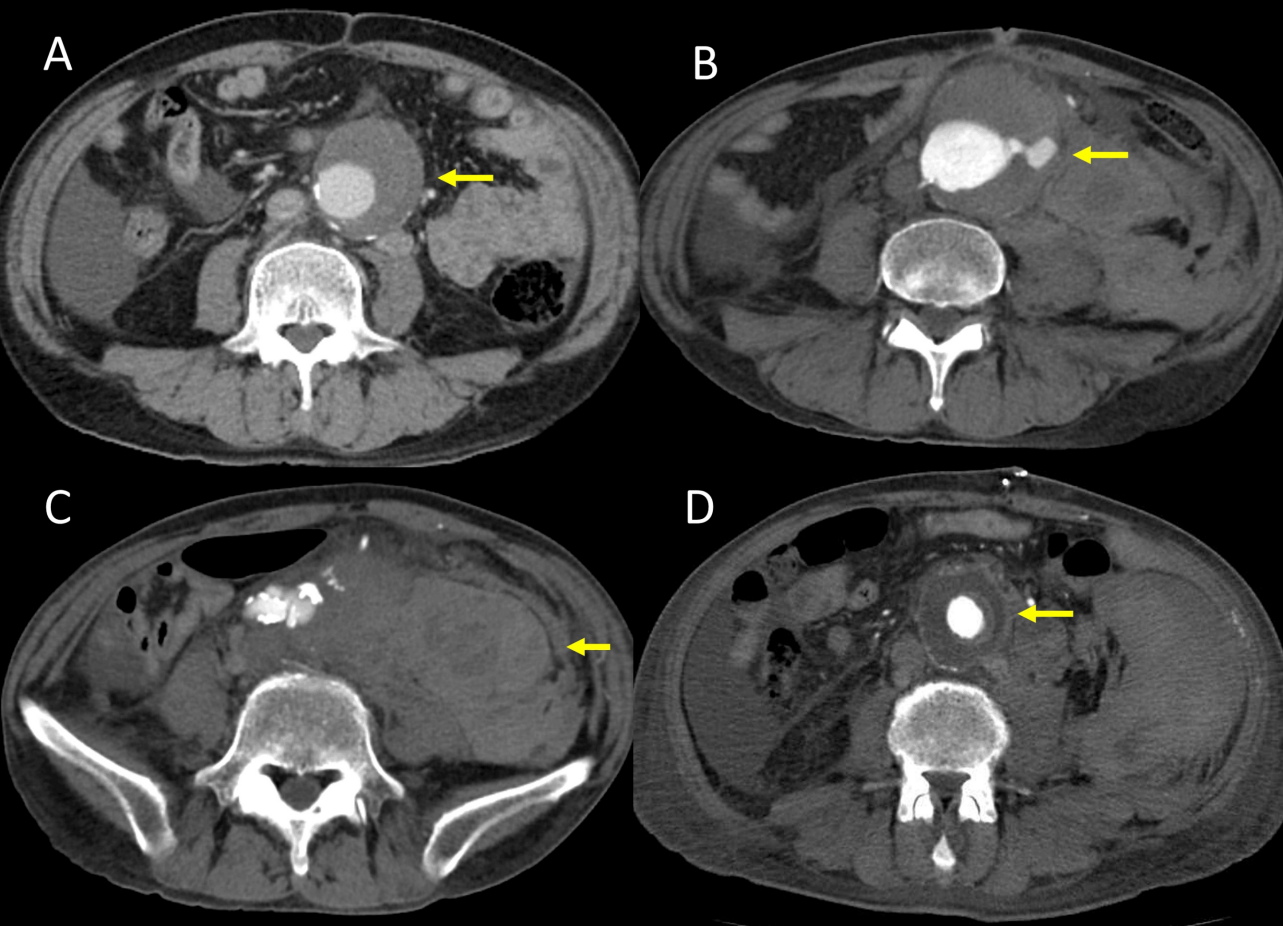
Comparison of pre-treatment and post-rupture axial CT findings. (A) Pre-treatment axial CT image showing an infrarenal abdominal aortic aneurysm measuring 52 mm. (B) Axial CT image after rupture demonstrating an enlarged aneurysm measuring 70 mm. (C) Axial CT image after rupture revealing a large retroperitoneal hematoma.
